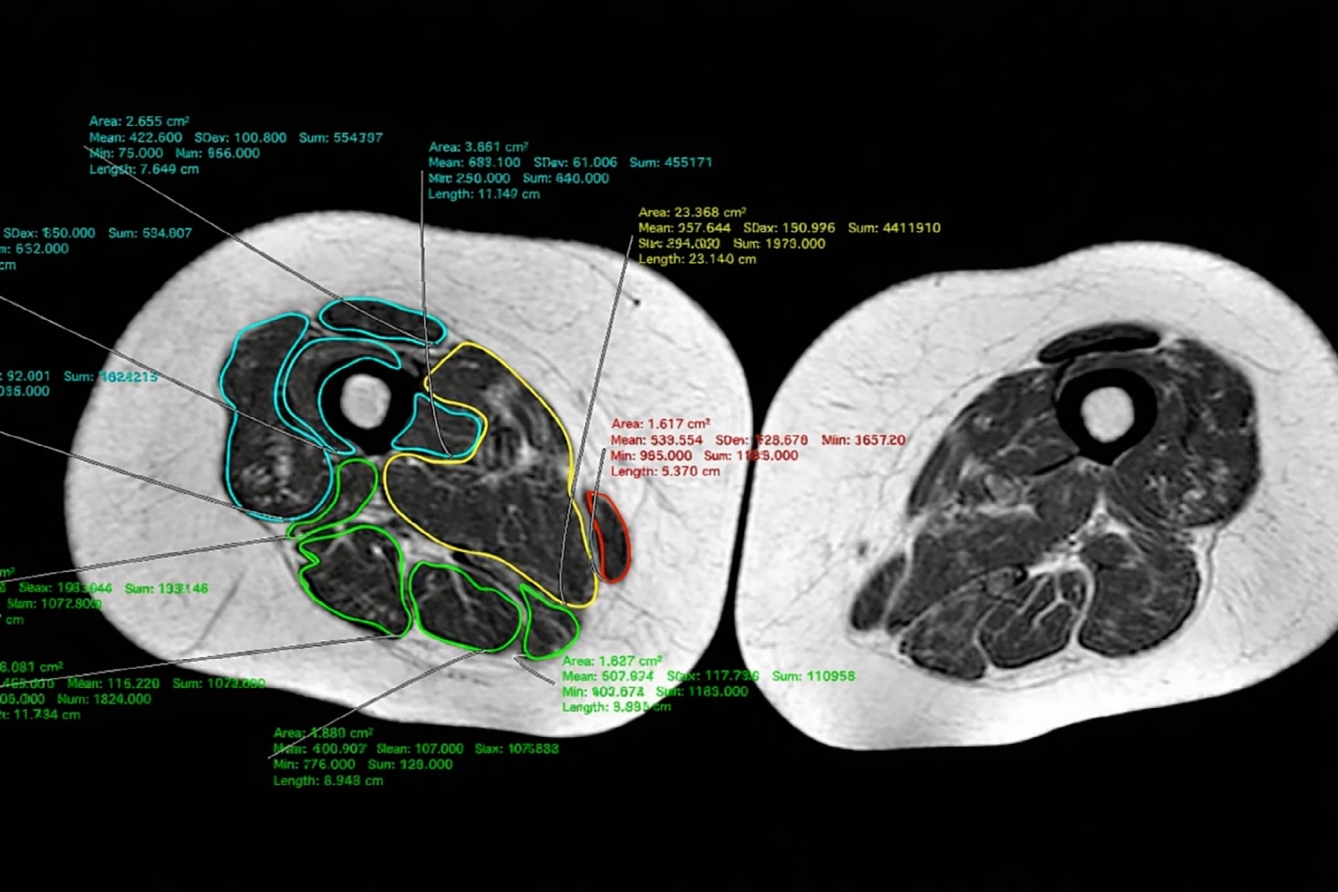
Sarcopenia assessment using magnetic resonance imaging (MRI). Example of segmentation of the mid‐thigh muscles on a single MRI image in a sarcopenic patient using the software Horos.
